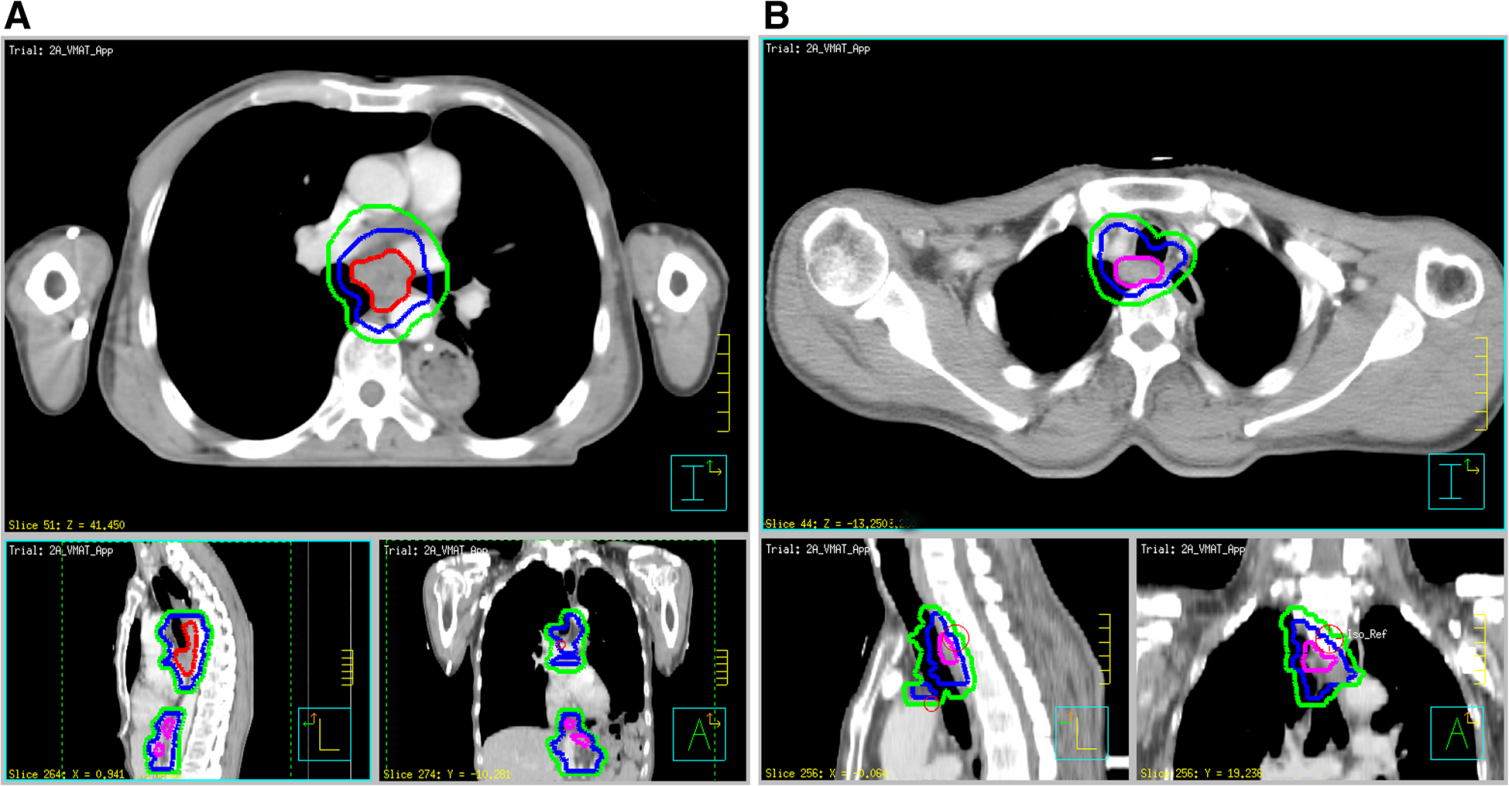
Examples of target volumes delineated in involved field irradiation (IFI) pattern are shown based on the planning computed tomography (CT) scans. a Lesions which were > 5 cm and far from the thoracic stomach recurred in Station 7, 8 M/L and 15–17. The IFI field includes Station partial 4R/L, 7, 8 U/M, and 15–17. b Lesions adjacent to the thoracic stomach recurred in Station 2R and palliative CRT was performed because of its time-to-recurrence > 16 months. The IFI field only includes peritumor regions. Pink outlines GTV-N, Red outlines GTV-T, blue outlines CTV, and green outlines PTV
